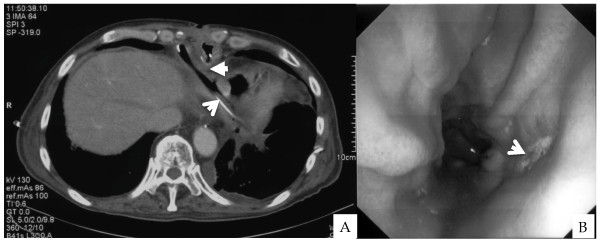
Post-operative CT shows pericardial drainage tube, indicated by an arrow, and muscular flap behind gastric tube, indicated by a triangular arrow (A); Postoperative upper GI endoscopy shows the healing ulcer, indicated by an arrow (B).
